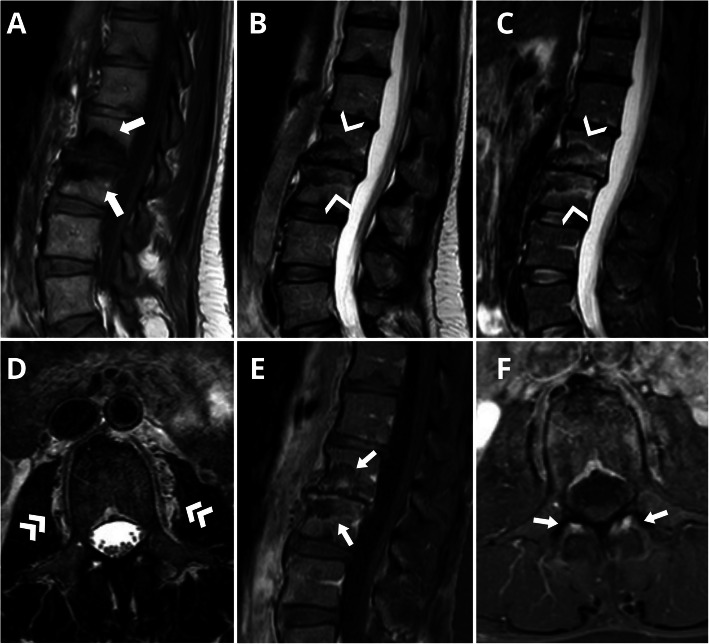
The first lumbar MRI. The sagittal T1WI (a) and the sagittal T2WI (b) show irregularities and lesions with marked hypointensity of the L2-–L3 vertebral body endplates, representing a loss of normal bone marrow signal on the T1WI (simple arrows) and sclerosis on the T2WI (open arrows). There is a slight retrolisthesis of the L2 vertebral body, suggesting some grade of instability. The gross morphology and height of the involved vertebral bodies are still preserved. There are associated degenerative changes with marginal osteophytes, important reduction of the L2–L3 intervertebral disc height and its T2WI signal, depicting disc dehydration, findings also observed at other levels of the thoracolumbar spine. The sagittal STIR-WI (c) demonstrate hyperintensity at the borders of the lesions, indicating oedema, a finding also observed on the T2WI in a subtler way (open arrows). The axial T2WI (d) show an enlargement of paravertebral surrounding soft tissues, suggestive of inflammation (double arrows). The sagittal (e) and axial (f) gadolinium-enhanced fat sat T1WI demonstrate subtle marginal enhancement of the vertebral body lesions and of the areas adjacent to interapophyseal articulations and spinous processes, from L2–L3 to L5–S1, compatible with the involvement of both anterior and posterior elements (simple arrows). The paravertebral enlarged soft tissues are also enhanced
